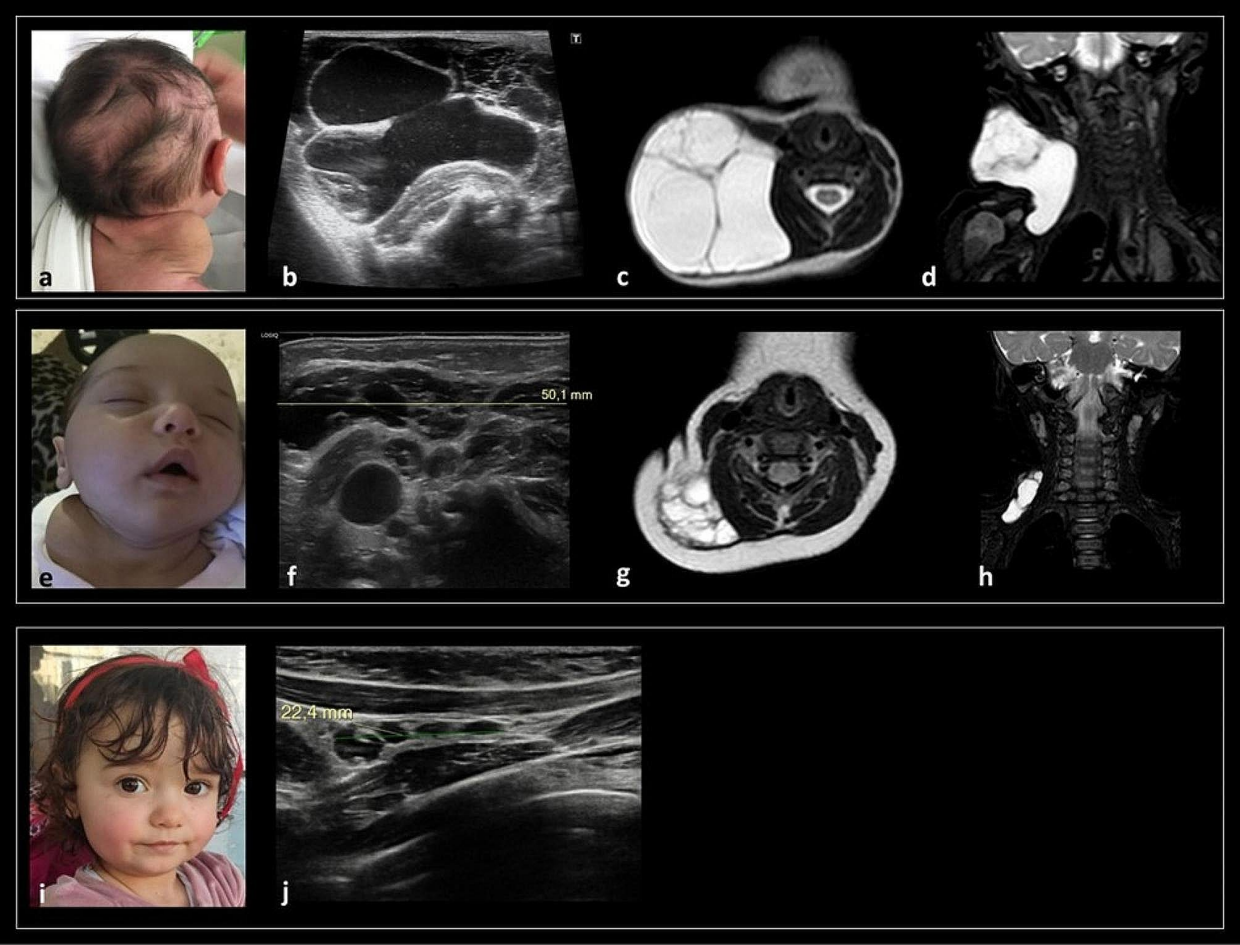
(a) Clinical examination at 8 DOL showing a cervical swelling mass, extending from the posterior lateral area of the neck to the ipsilateral supraclavicular region. Pre-treatment US (b) and MRI (c, d - axial and coronal T2-w respectively) confirmed a lateral cervical multiloculated cystic mass in the posterior lateral triangle of the neck, extending inferiorly in the right infraclavicular space, without involvement of mediastinum and visceral space of the neck. One-month post-sclerotherapy, the patient presented a marked clinical reduction of the cervical mass (e). US examination confirmed a dimensional reduction of the lymphatic lesion, with maximal extension of 5 cm towards the deep tissues (f). Six months post-sclerotherapy, MRI (g, h - axial and coronal T2-w respectively) showed > 50% volumetric reduction with a residual macrocystic mass in the right posterior lateral neck, without infiltration of muscles and deep neck spaces. (i) Picture at last clinical evaluation (2 years and 2 months of age) did not document any visible mass, and US examination (j) showed resolution, with a residual hypo-/anechoic multiloculated component in the right supraclavicular area (24 × 5 mm)
